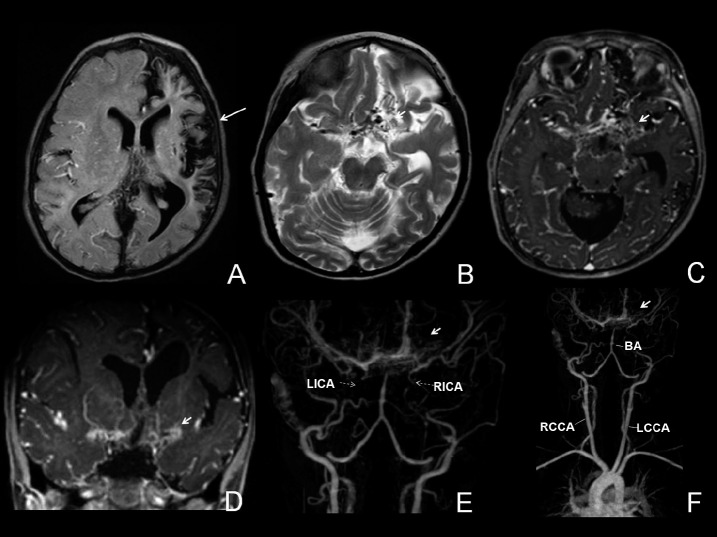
Magnetic resonance imaging performed with Philips 3.0 Tesla apparatus showing FLAIR (fluid-attenuated inversion recovery) images (A), T2 (B), post-contrast T1 (C and D) and angioresonance of intracranial and cervical vessels (E and F). Asymmetry of the brain hemisphere can be seen in A, with marked atrophy on the left, distortion of the gyri and prominence of the sulci, involving the cortex and the left frontotemporal-parietal white matter, while sparing the ipsilateral occipital region (large arrow). B, C and D show exuberant tortuous collateral circulation above the occlusion of the supraclinoid internal carotids. E and F show occlusion of the internal carotids in the supraclinoid region and above the basilar artery, associated with exuberant and tortuous collateral circulation in perforating vessels (dotted and small arrows).
